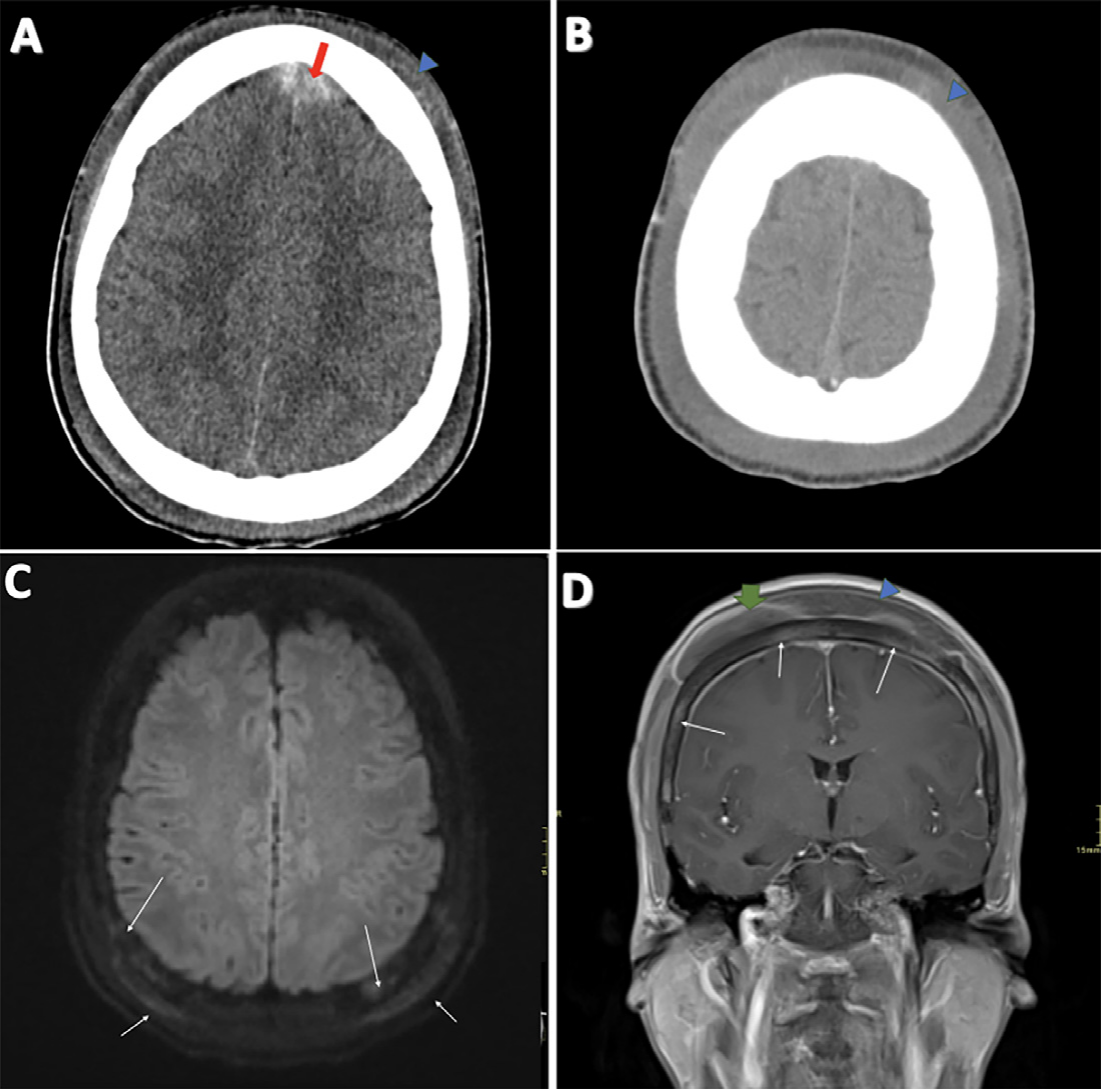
(A) Axial CT subdural window: The long, thick red arrow points to the extra-axial epidural collection. The blue arrowhead indicates subgaleal soft tissue edema. (B) Axial CT soft tissue window: The blue arrowhead indicates subgaleal soft tissue edema, appearing isodense on the axial CT soft tissue window. (C) Axial Diffusion-Weighted Imaging (DWI): The thin, long white arrows mark a region of occipital osteonecrosis. The thin, short white arrows point to the corresponding adjacent subgaleal hemorrhage. (D) Coronal T1WI Post-Contrast: The long, thin white arrows indicate calvarial heterogeneity with enhancement, suggesting areas of presumed bone infarction. The short, thick green arrows highlight pockets of subgaleal peripherally-enhancing collections with intrinsic T1 hyperintense signals. The blue arrowhead marks subgaleal soft tissue edema.
